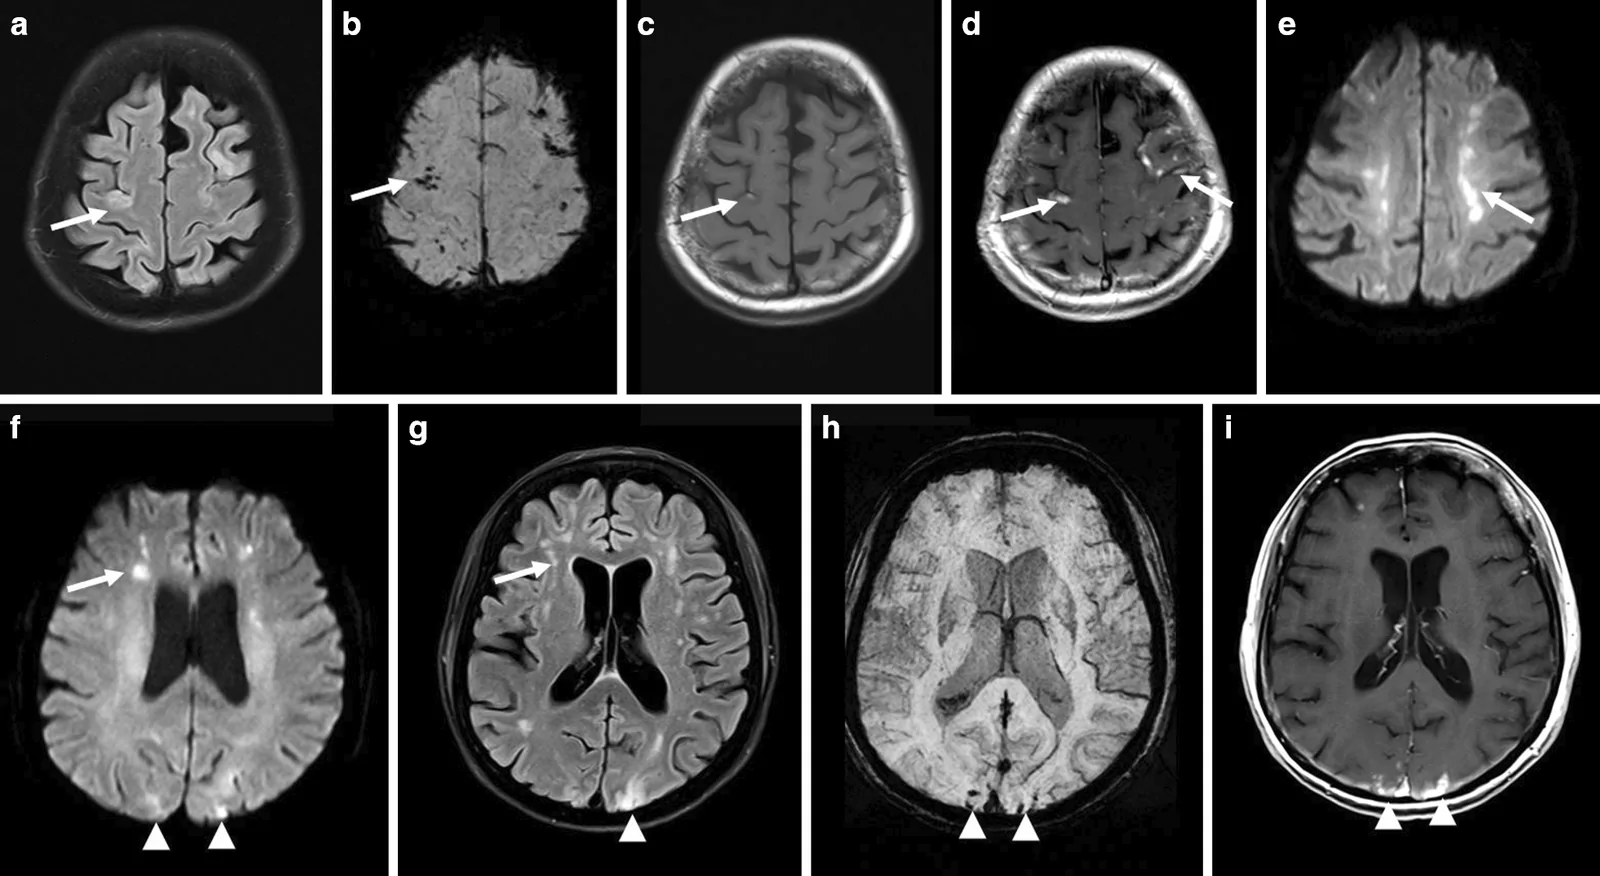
Eosinophilic granulomatosis with polyangiitis (EGPA; Churg-Strauss syndrome) in a 65-year old woman suffering from progressive amnestic syndrome and slight right-sided hemiparesis. a–e bilateral cortical and subcortical hyperintense lesions (a, g arrow; fluid attenuated inversion recovery (FLAIR) images) with punctuate signal loss (b arrow; susceptibility weighted imaging (SWI)) and hyperintense signal on T1 WI (c arrow), inhomogeneous enhancement on post contrast (pc) T1 WI (d arrow); bilateral diffusion restriction on (e, f arrow; DWI, b = 1000 s/mm^2^); f–i additional occipital sulcal conglomerations (f–i arrowhead) with distinct contrast enhancement (i , arrowhead), signal loss on SWI (h arrowhead), diffusion restriction (f arrowhead) and hyperintense signal on FLAIR images (g arrowhead)
